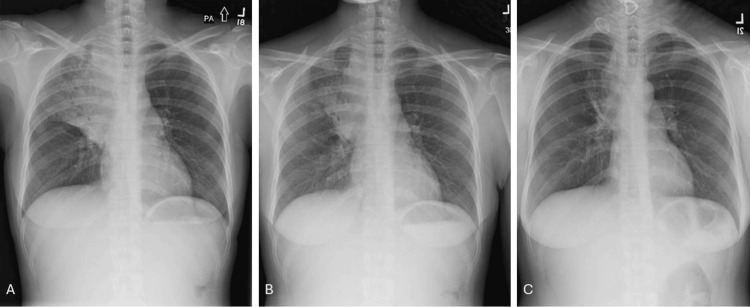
A: Chest radiograph (PA view) on admission shows right upper lobe consolidation. B: Chest radiograph (PA view) one month after starting MAC therapy. C: Chest radiograph after completion of therapy.
